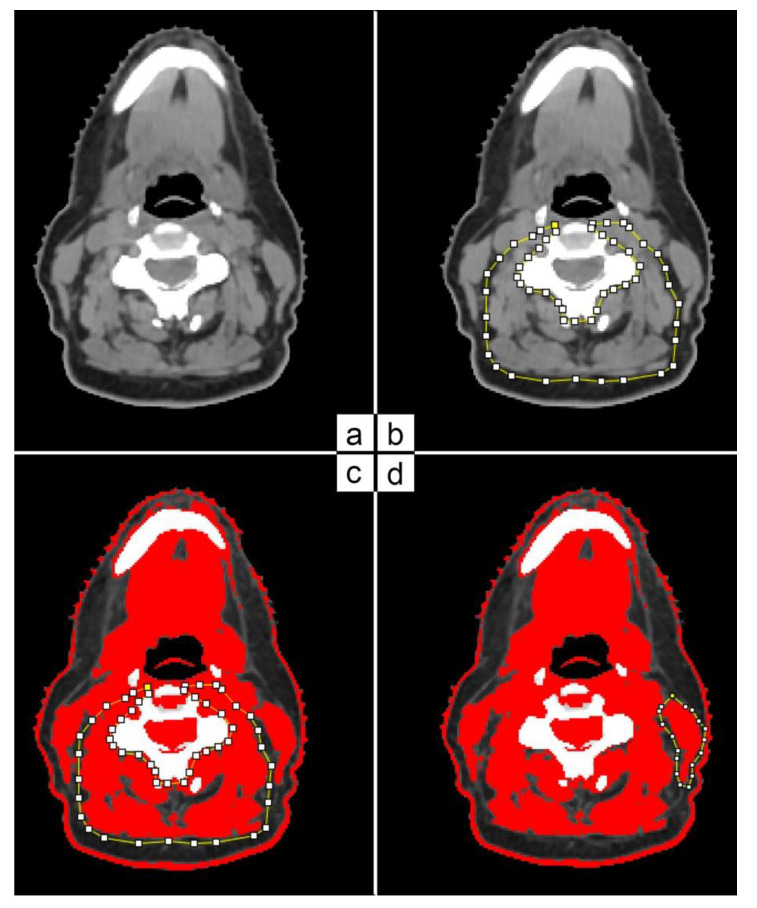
C3 paravertebral and SCM area delineation on CT scan based on HU threshold.
